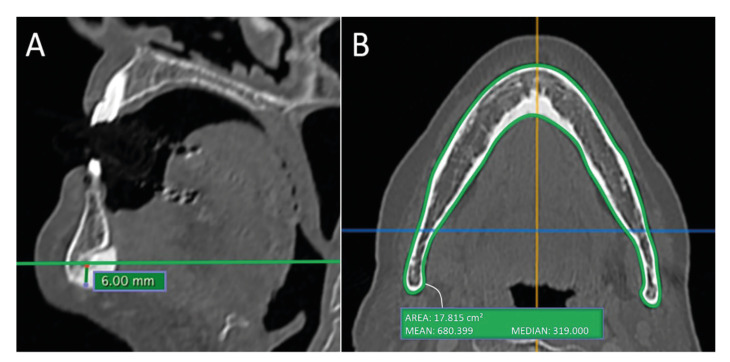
A) Computed tomography (CT) measurement method, 6 mm above the base of the mandible (sagittal image). B) Region of interest (ROI) was set on the mandibular body regions contour including medullary and buccolingual cortical bones - mean and median CT grayscale values (axial image).
